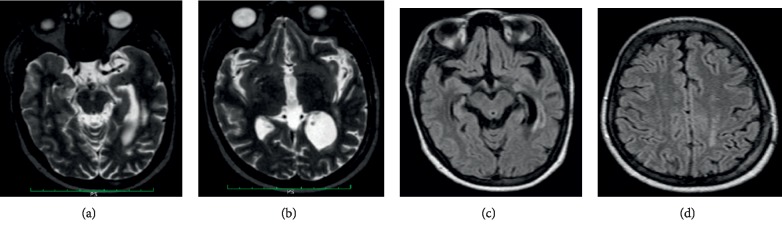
MRI brain scan performed three months after evacuation of the extradural hematoma. (a) Axial T2-weighted MRI scan showing multifocal hyperintensities on the left periventricular area and dilated posterior horn of the left lateral ventricle. (b) Coronal T2-weighted MRI scan and (c) axial “fluid attenuated inversion recovery (FLAIR)-MRI. FLAIR-MRI are used to enhance T2-weighted scans to identify hyperintense lesions by suppressing cerebrospinal fluid. Both images (b and c) show prominent left sylvian fissure and dilated posterior horn of the left lateral ventricle and left temporal lobe atrophy. (d) Axial FLAIR-MRI showing white matter hyperintensity of the left parietal lobe signal changes on the parietal bone.
